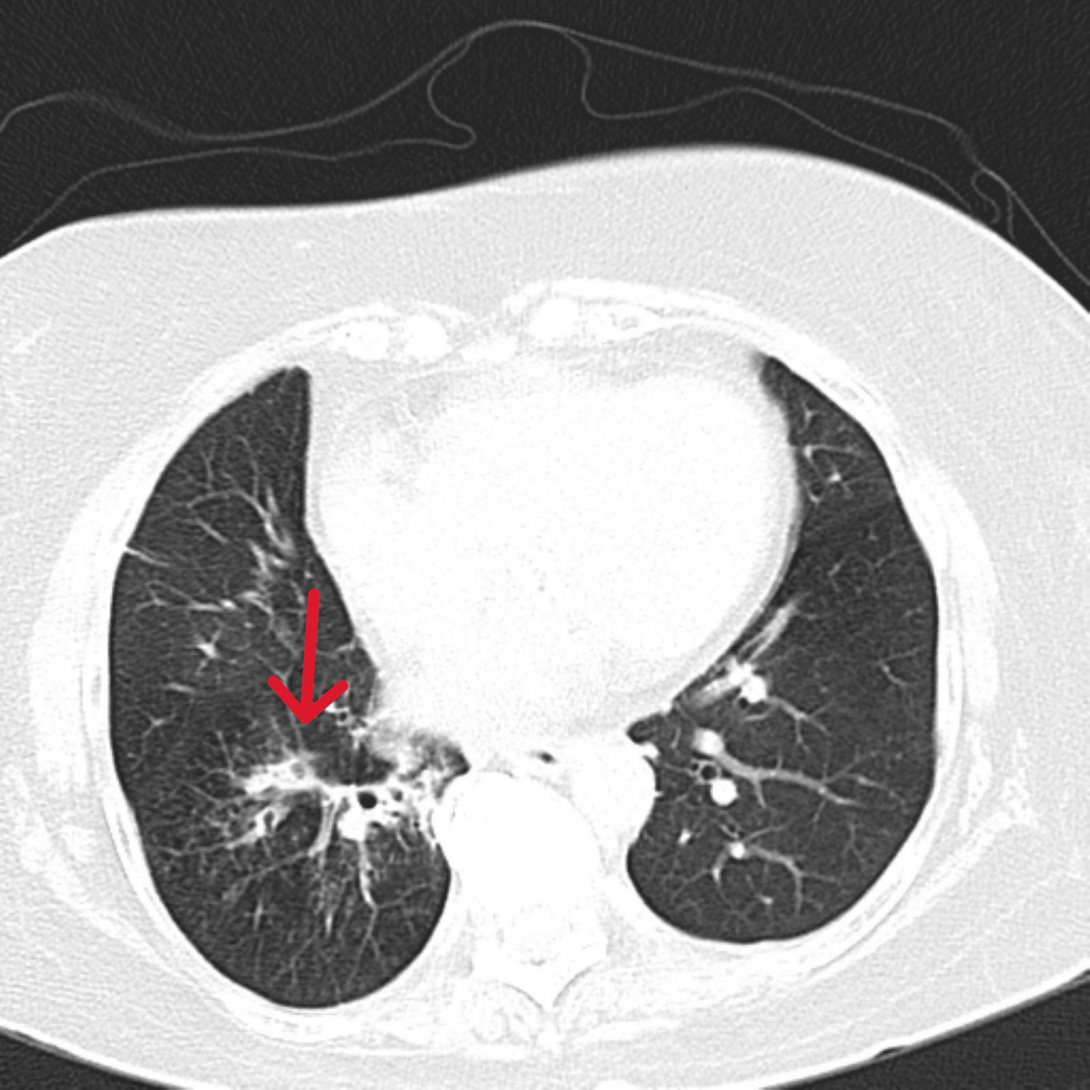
Axial section of a CT Chest Case 2: Patchy consolidation (red arrow) with cystic bronchiectasis in posterior and medial basal segments of right lower lobe.
